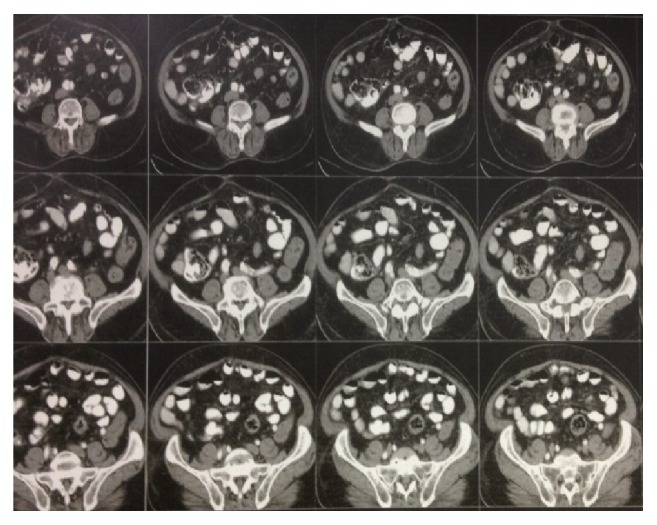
CT scan 5 months before surgery.
